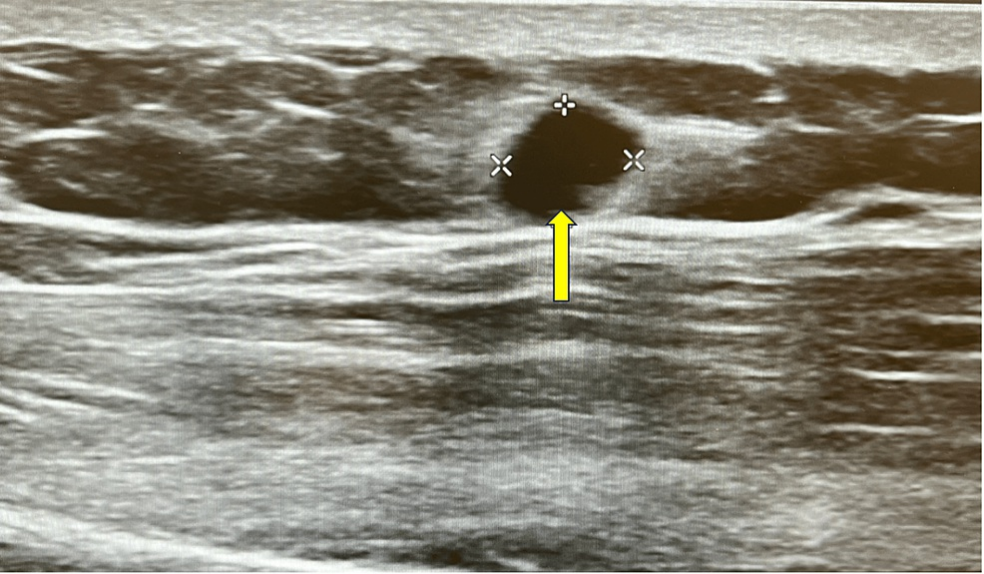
B-mode or gray-scale ultrasound image of the left proximal arm shows a 5×5-mm hypoechoic mass. The yellow arrow highlights the hypoechoic mass found on the gray-scale ultrasound.
